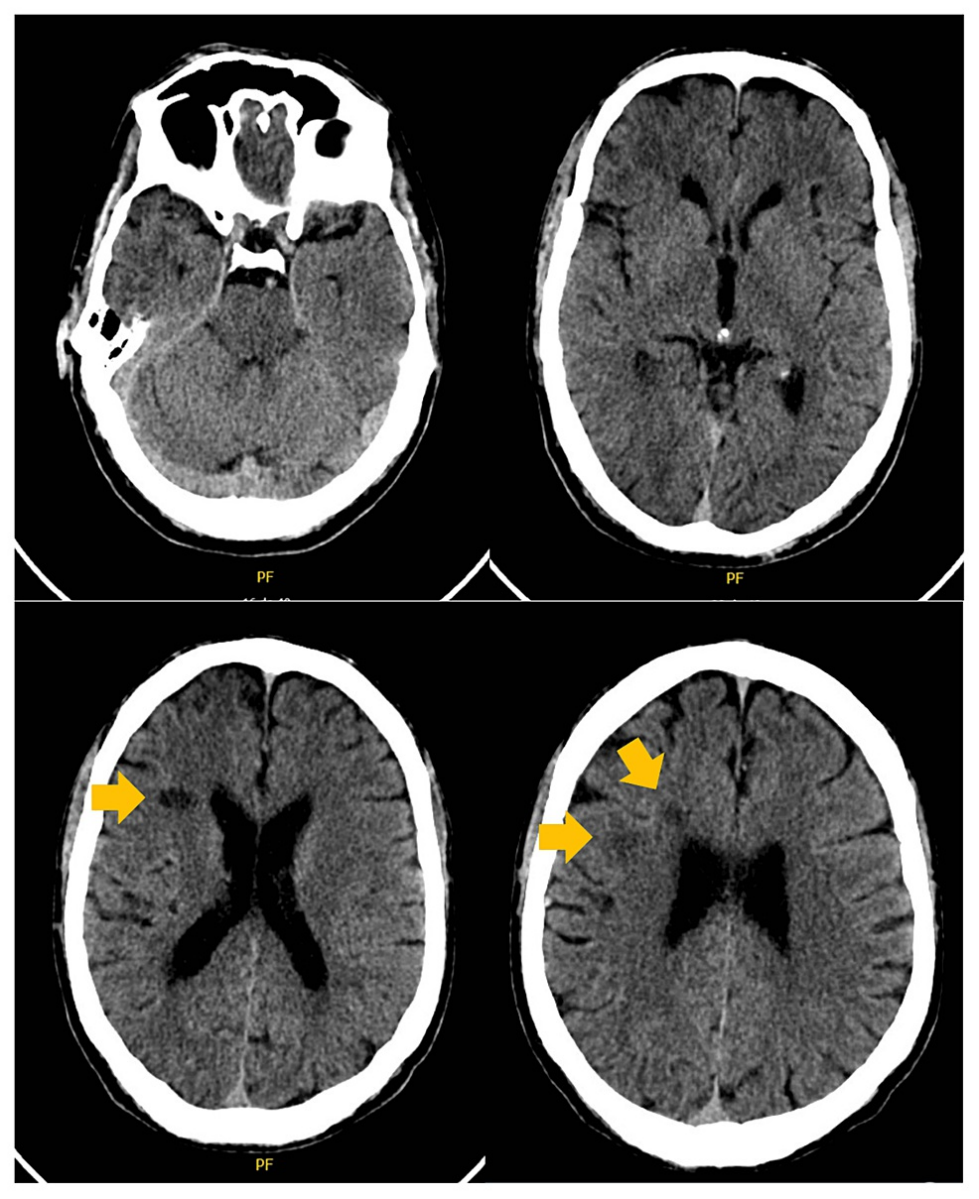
A brain CT scan without contrast showed multiple subcortical hypodense lesions without mass effect, compatible with the past medical history of multiple sclerosis. No mass lesions, hemorrhage, hydrocephalus, or extra-axial collections were found.
